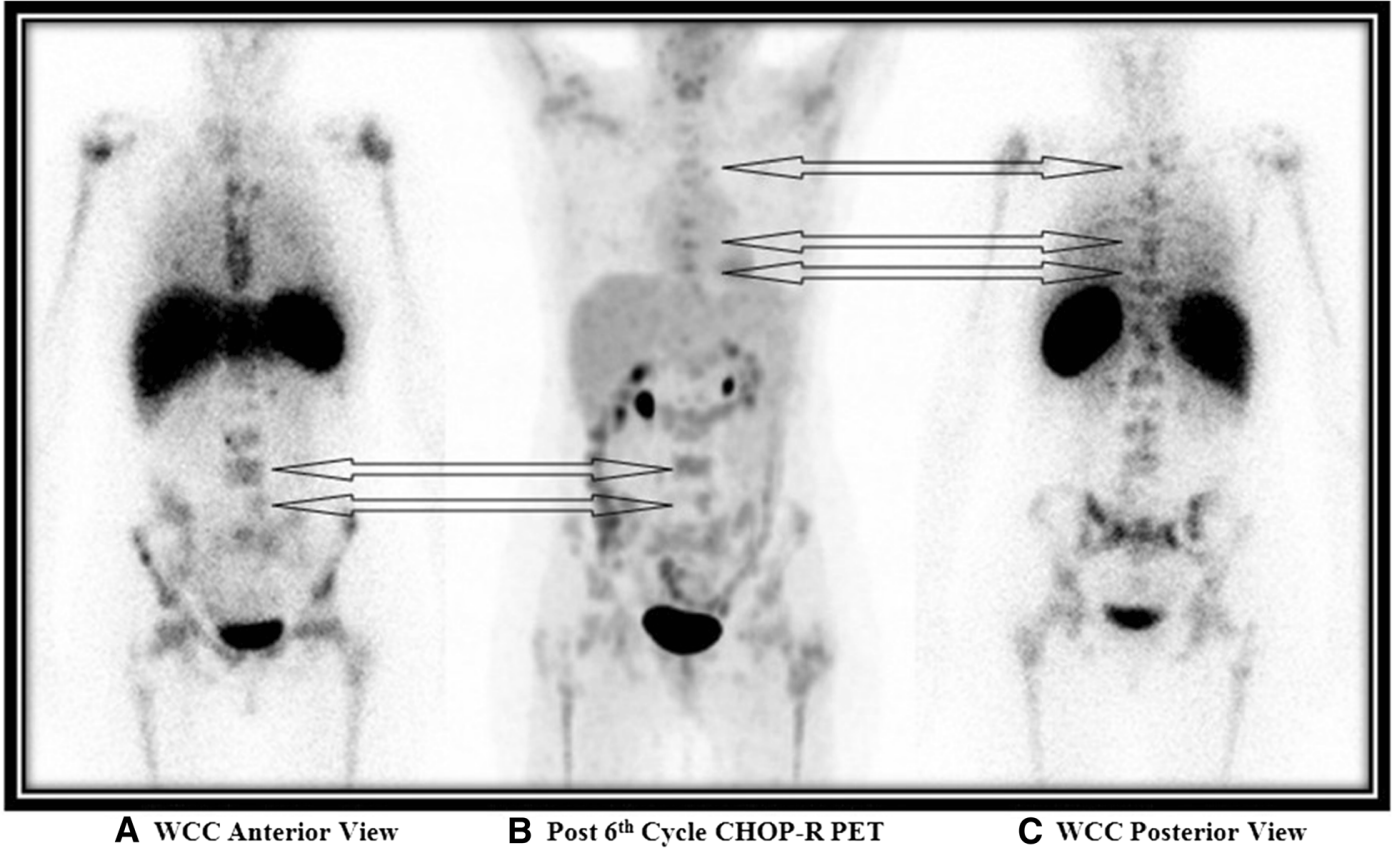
Comparison of ^18^F-fluorodexoyglucose positron emission tomography scan uptake in spine with sites of normal bone marrow activity on whole body white cell scan post six cycles of chemotherapy. White cell scan anterior planar view (A), White cell scan posterior planar view (C) three-dimensional maximal intensity projection (MIP) ^18^F-FDG PET scan images (B). The overall pattern of FDG uptake on PET scan is similar to the white cell scan uptake in keeping with sites of normal regenerating marrow rather than lymphoma. Arrows highlight similarities in FDG and white cell uptake in the thoracic and lumbar spine.
